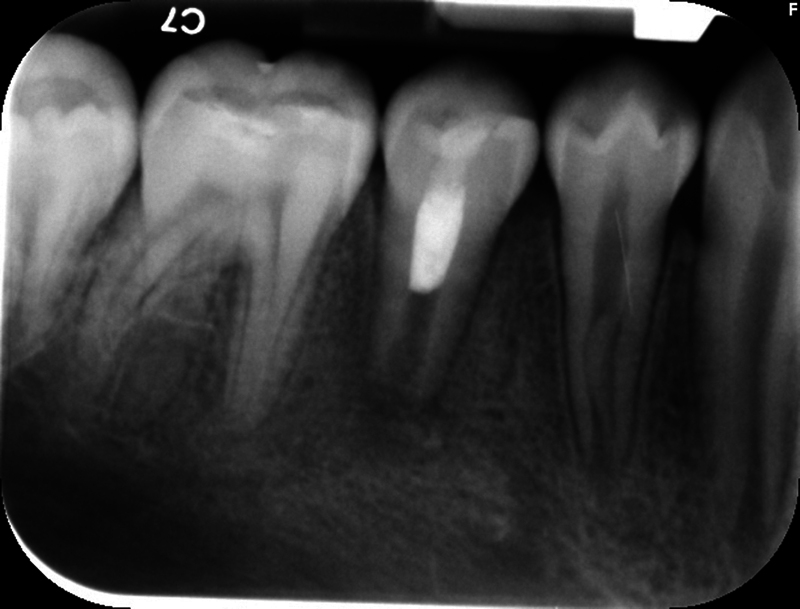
Immediate postoperative periapical radiograph of tooth #29.
